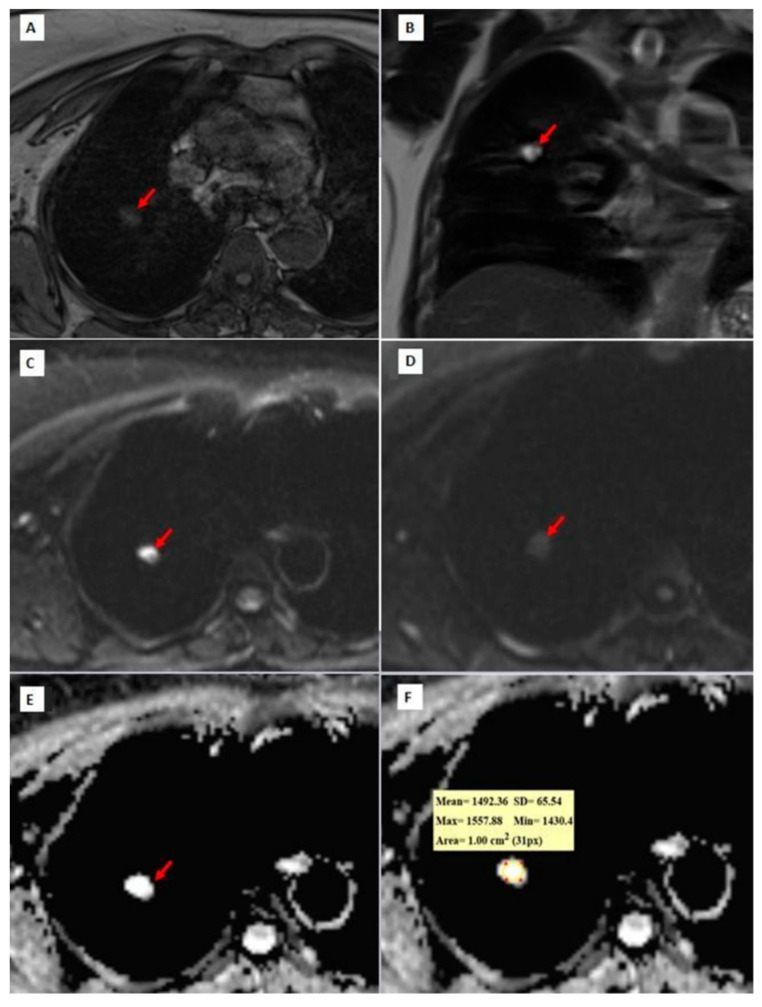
A 72-year-old patient diagnosed with grade 1 adenocarcinoma. (A) An axial T1W image showing a hypo-isointense nodule with lobulated contour at the right upper lobe (red arrow). (B) A coronal T2W MRI image showing a hyperintense right upper lobe lung nodule (red arrow); (C,D) b = 0 and b = 800 DW images are shown, respectively. In the b = 0 DW image, there is a hyperintense nodule in the upper lobe of the right lung. In the b = 800 DW images, it is seen that the nodule has lost its signal significantly. (E,F) In the ADC map, the mean ADC value is measured as 1492.36 × 10^−6^ mm^2^/s within the lesion.
